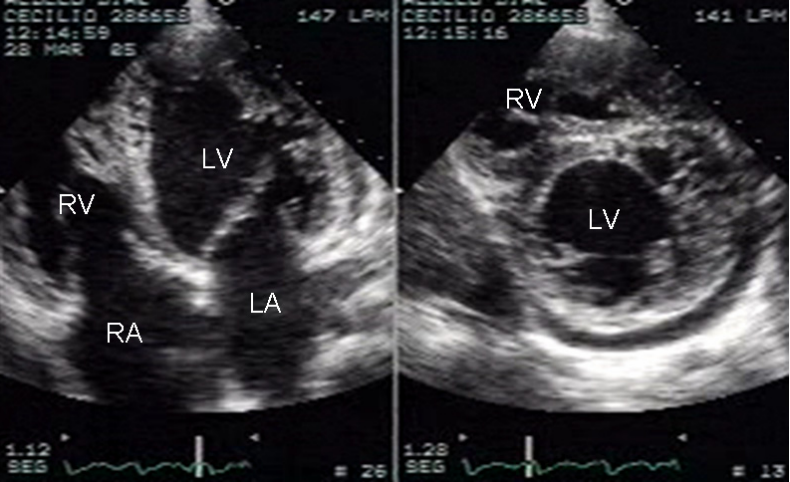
Two-dimensional apical four chamber and parasternal short axis images at the level of the ventricles show dilatation of both ventricles, multiple trabeculae and intertrabecular recesses in inferior, lateral, anterior walls, middle and apical portions of the septum and apex of the left ventricle. A mild pericardial effusion can be observed. LV: Left ventricle; LA: Left atrium; RV: Right ventricle; RA: Right atrium.
